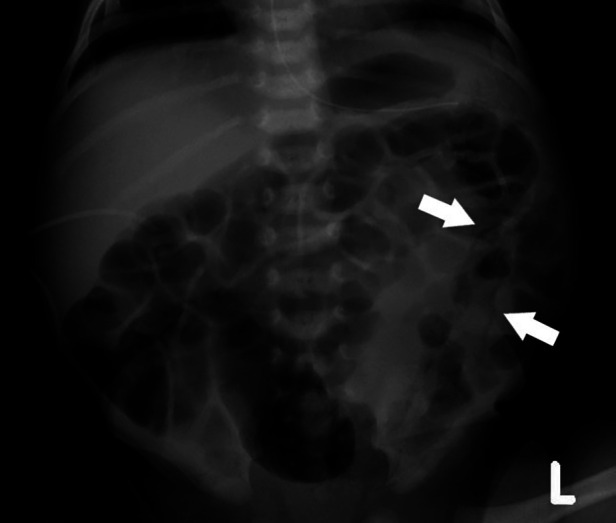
Abdominal x-ray demonstrating pneumatosis intestinalis.
